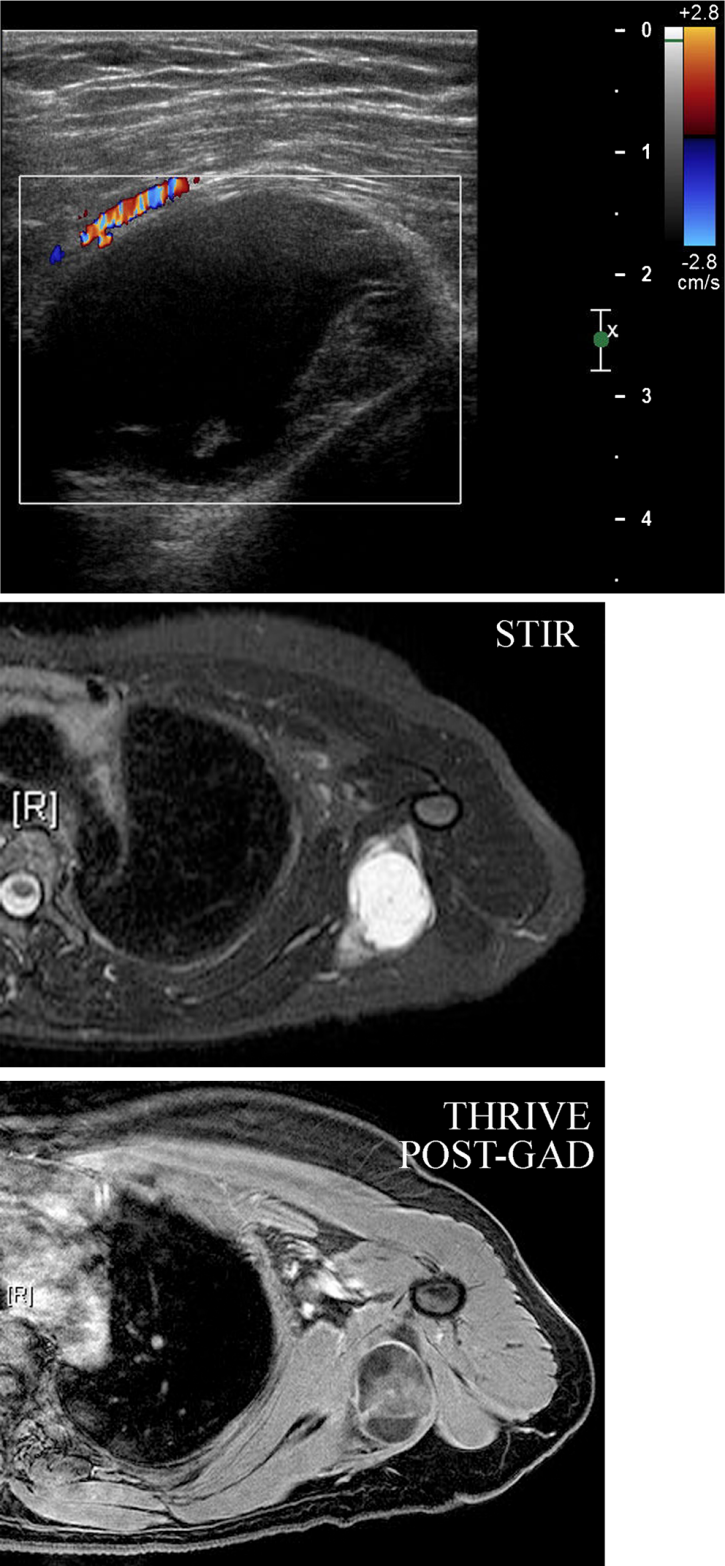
A 43-year-old female presents with a stable left axillary mass for 3–4 years, which the patient reports to fluctuate with her menstrual cycle. MLO and CC views reveal no focal mammographic abnormality (not shown here). b Ultrasound demonstrated a complex oval mass with cystic and solid components lying within the muscle. Colour Doppler analysis demonstrates some peripheral blood flow. c Axial STIR and d axial post-gadolinium sequences demonstrate an ovoid lesion within the latissmus dorsi muscle at the level of the axilla, which was hypointense to muscle on T1-weighted images (not shown here) and shows increased signal intensity on the fluid-sensitive STIR sequence shown here. Post-gadolinium imaging shows some internal enhancement. CT-guided needle biopsy was performed. Final diagnosis: intramuscular myxoma of the latissimus dorsi
